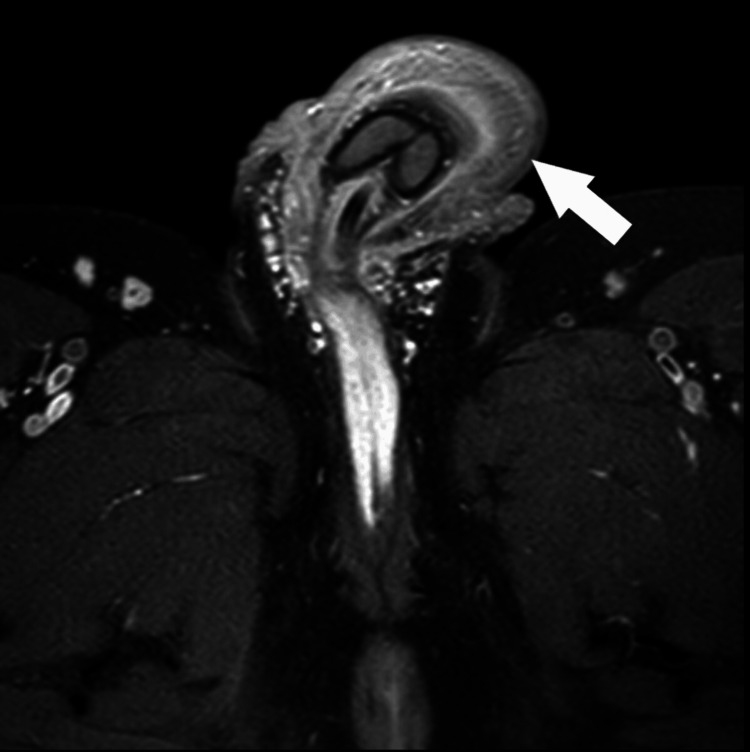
Axial plane MRI image showing soft tissue swelling
